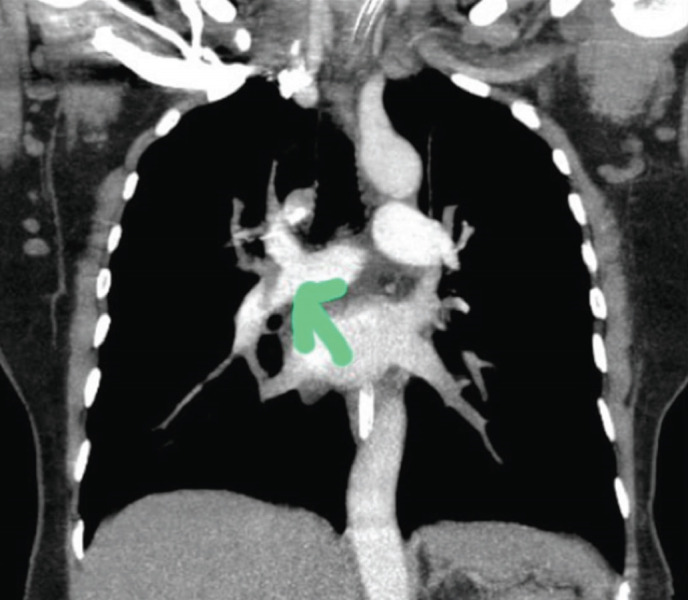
CT pulmonary image showing filling defect in the right pulmonary artery and branches (shown by green arrow).
